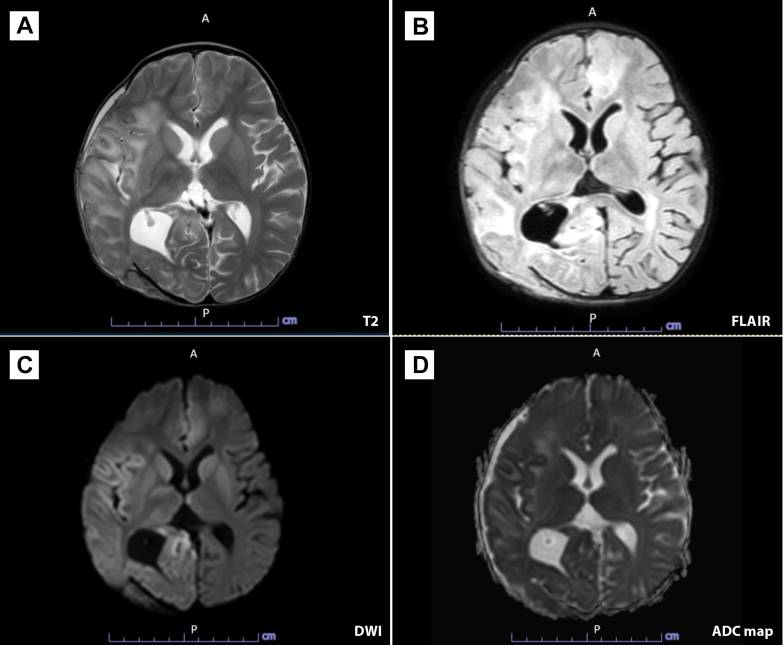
Magnetic Resonance Imaging Brain (Day 8) T2-weighted (T2) and fluid-attenuated inversion recovery (FLAIR) images (A and B) demonstrate hyperintense lesions involving the cortex and subcortical white matter of the right occipital, right parietotemporal, and left frontal lobes. Diffusion-weighted image (DWI) and apparent diffusion coefficient (ADC) map images (C and D) show cortical restricted diffusion consistent with acute infarction. The adjacent white matter demonstrates no diffusion restriction, suggestive of vasogenic edema.
